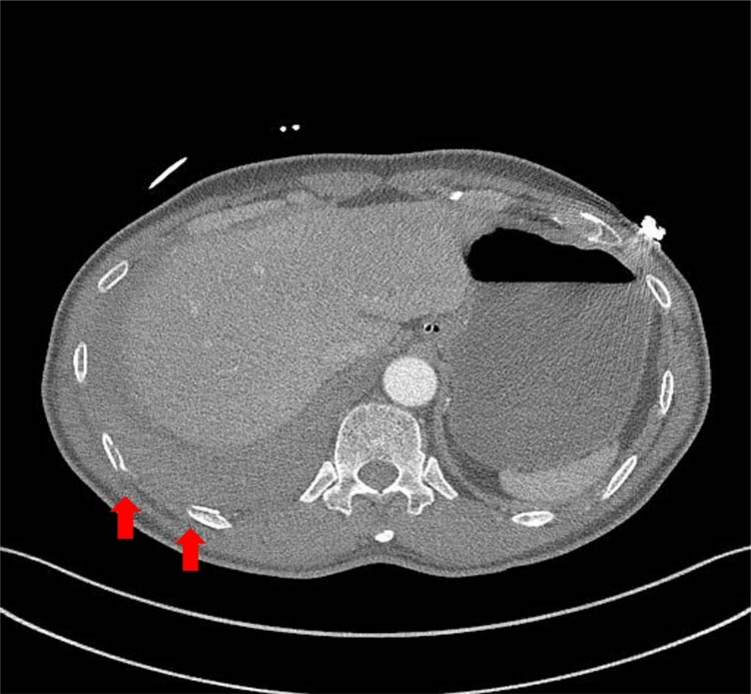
Chest contrast-enhanced CT showed massive hemothorax with multiple rib fractures including 10th, 11th ribs (arrow) with no active extravasation of contrast.
